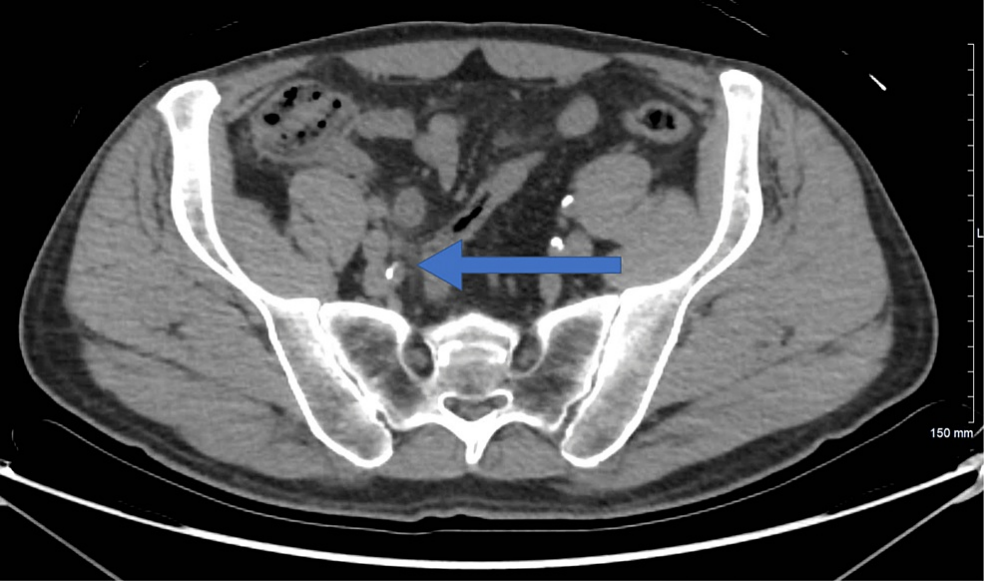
Axial computed tomography scan done on April 24th.
